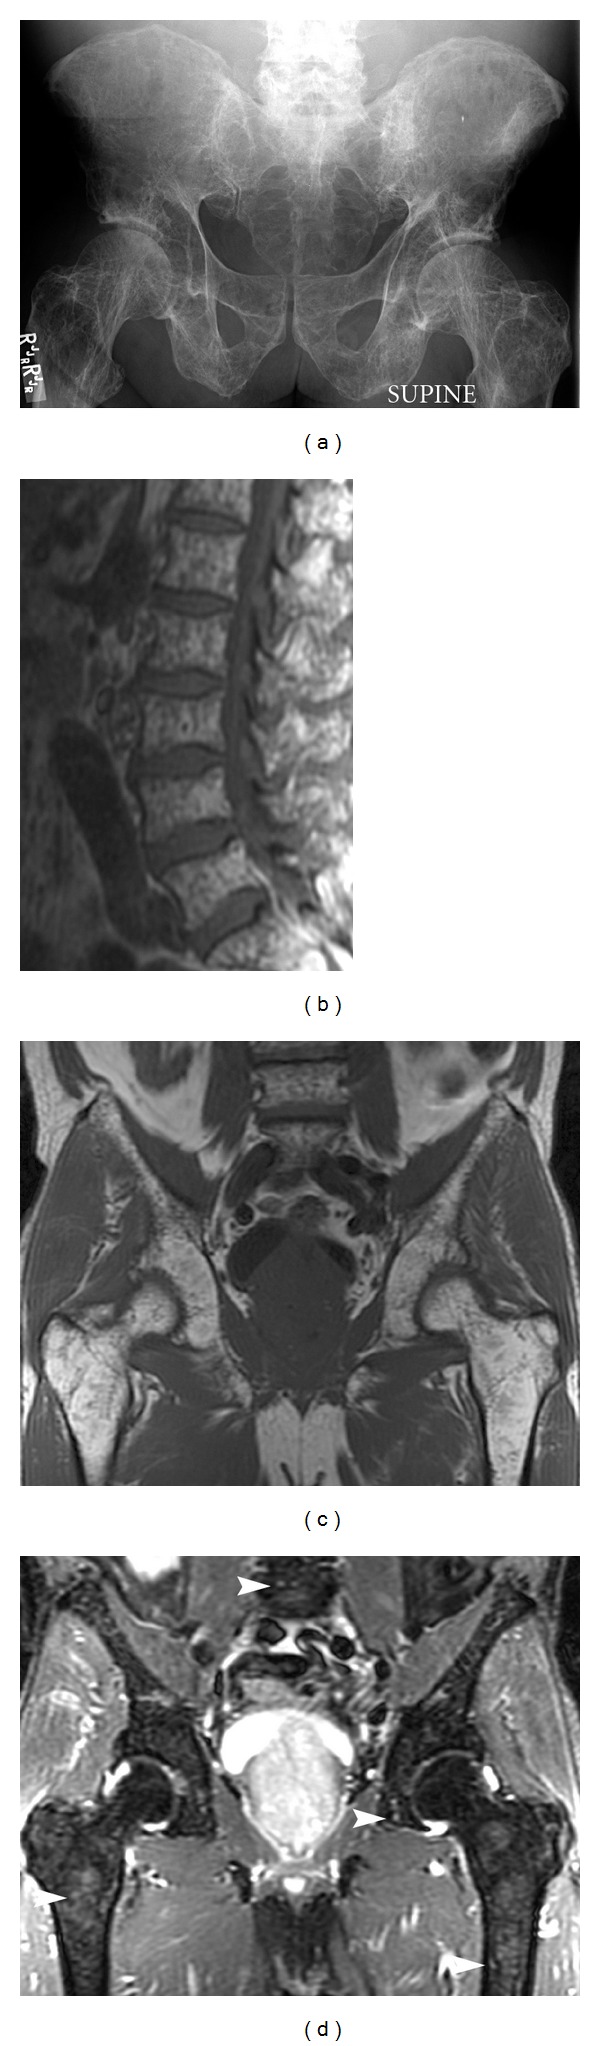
Multiple myeloma. Elderly patient with multiple myeloma. Plain film (a) demonstrates extensive osteopenia and multiple punched-out lytic lesions involving the entire pelvis. Sagittal T1 (b) of the lumbar spine, coronal T1 (c), and coronal STIR (d) demonstrate innumerable T1 hypointense and T2 hyperintense lesions (arrowheads) in the medullary cavity of the entire visualized skeleton.
